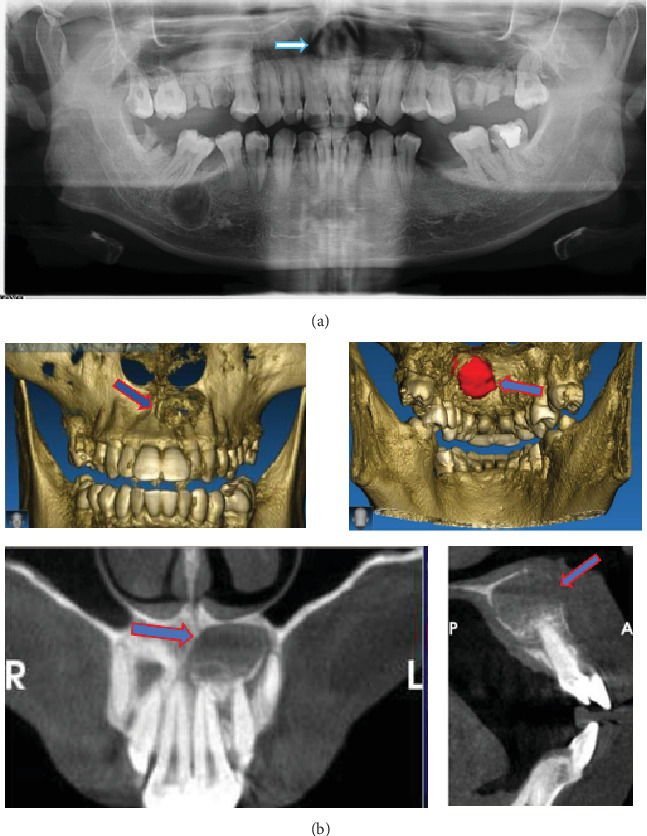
(a) Orthopantomogram X-ray feature. (b) CBCT of the anterior maxilla. The arrows indicate the location of the cyst area.
